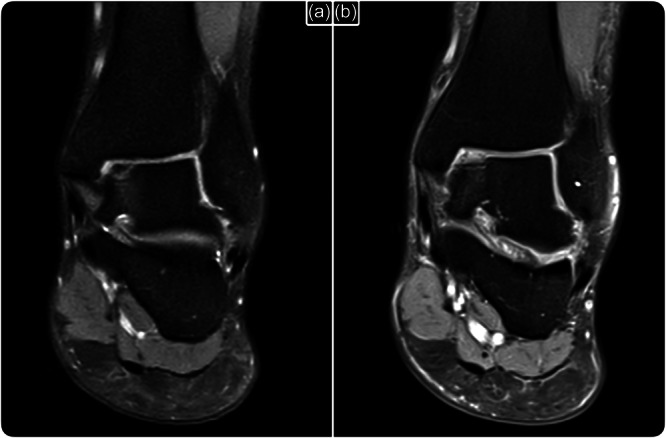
T2 MRI of a patient treated with the ACIC technique, preoperatively (a) and at 12 postoperatively (b), for an OLT located on the medial side. ACIC, autologous collagen‐induced chondrogenesis; MRI, magnetic resonance imaging; OLT, osteochondral lesions of the talus.
